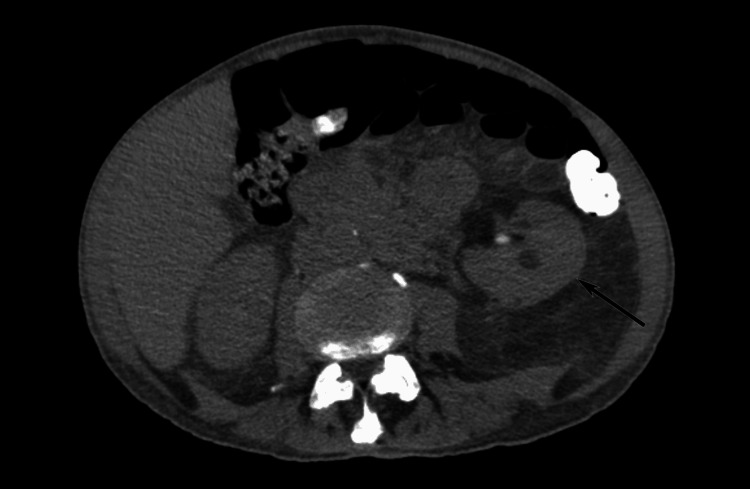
Supernumerary kidney Abdominal computed tomography (CT) scan without intravenous contrast medium. The supernumerary kidney is located on the left, caudally to the left normal kidney (black arrow).
